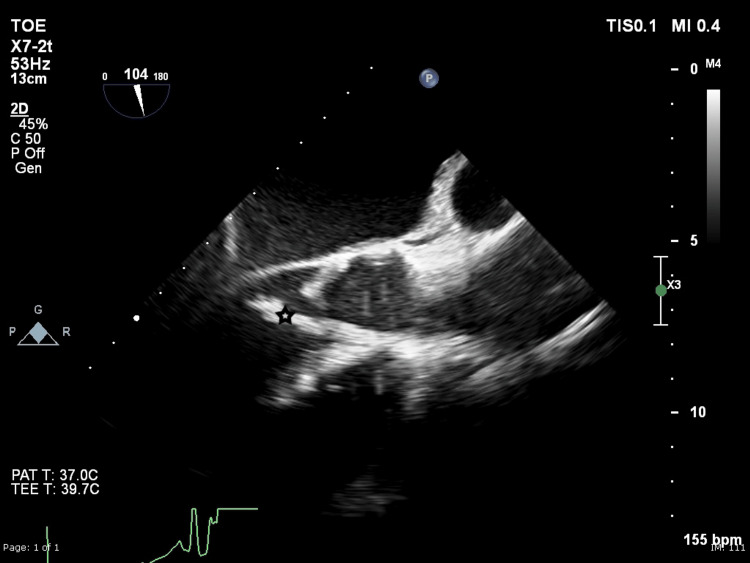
Intraoperative TOE shows a large aortic valve vegetation denoted by a star TOE: transesophageal echocardiogram
